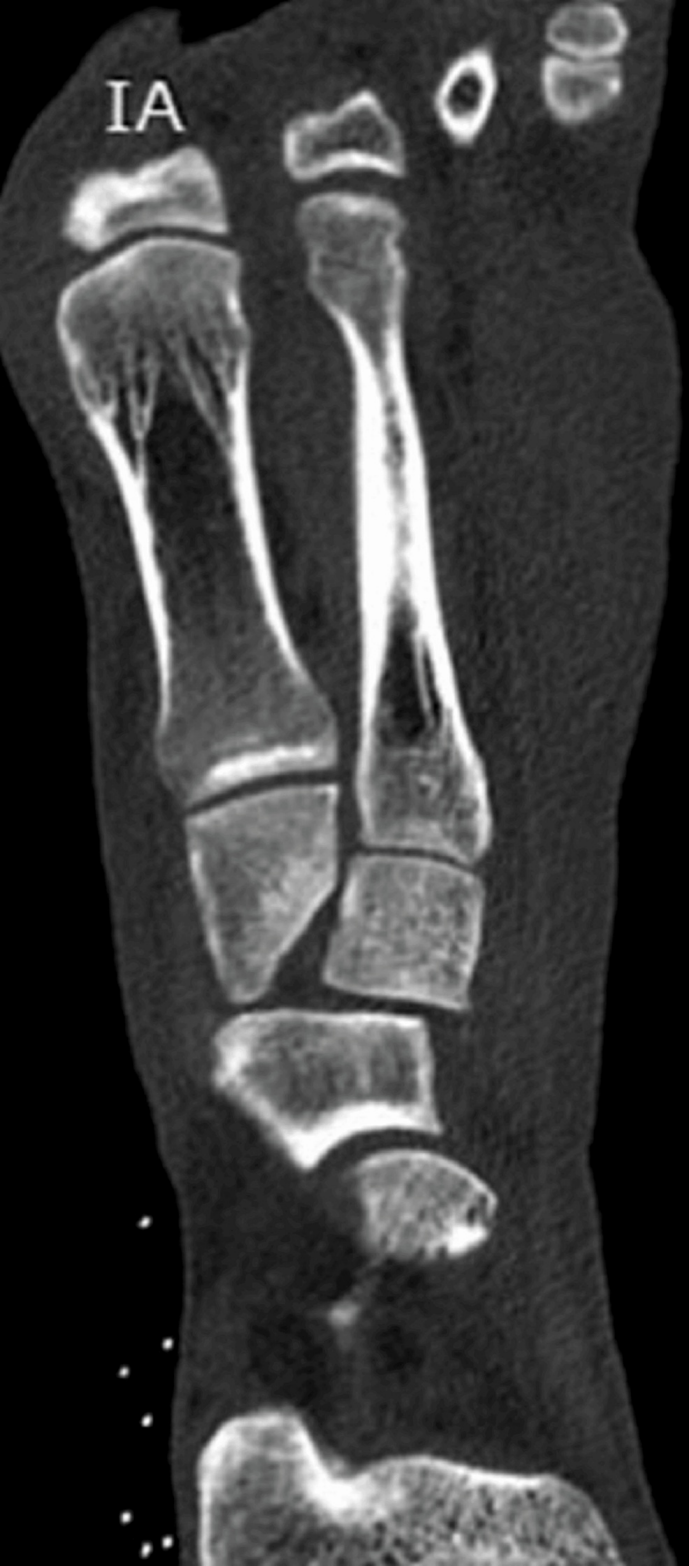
CT that shows Lisfranc joint without damage
